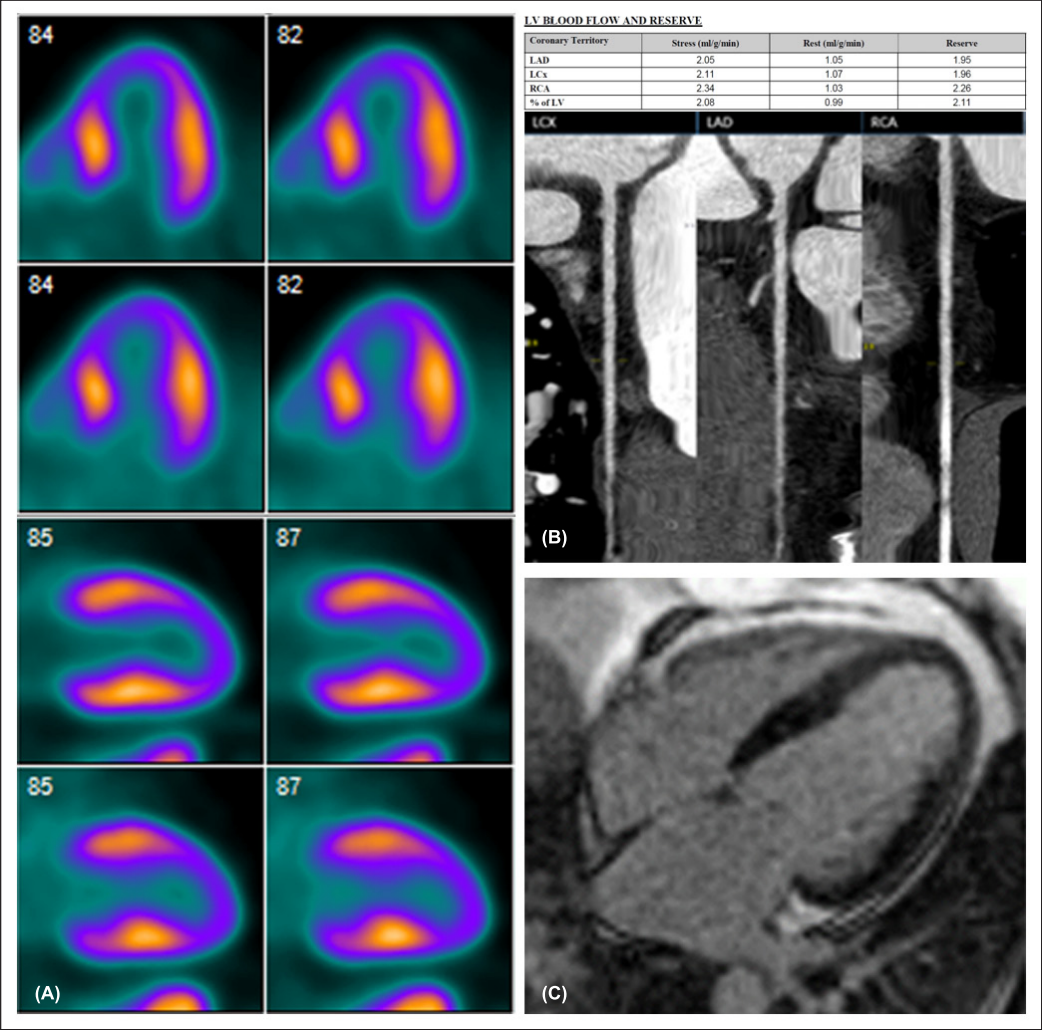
(A) Positron emission tomography cardiac images horizontal and vertical long axis shows a medium-sized severe fixed defect in the distal anterior, lateral, inferior, and septal segments and apex. (B) Cardiac computed tomography angiography 3-dimensional reconstruction shows coronary arteries without stenosis. Cardiac positron emission tomography myocardial blood flow/myocardial flow reserve (MFR) for the coronaries shows normal MFR. (C) Late gadolinium enhancement cardiac magnetic resonance imaging shows no evidence of myocardial infarction or scar.
